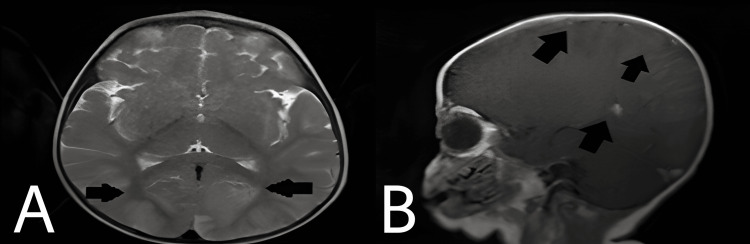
MRI of the brain shows symmetrical moderate-enhancing altered signal intensity cortex of right frontal and bilateral temporo-parieto-occipital regions (A) and in the splenium of corpus callosum (B).
